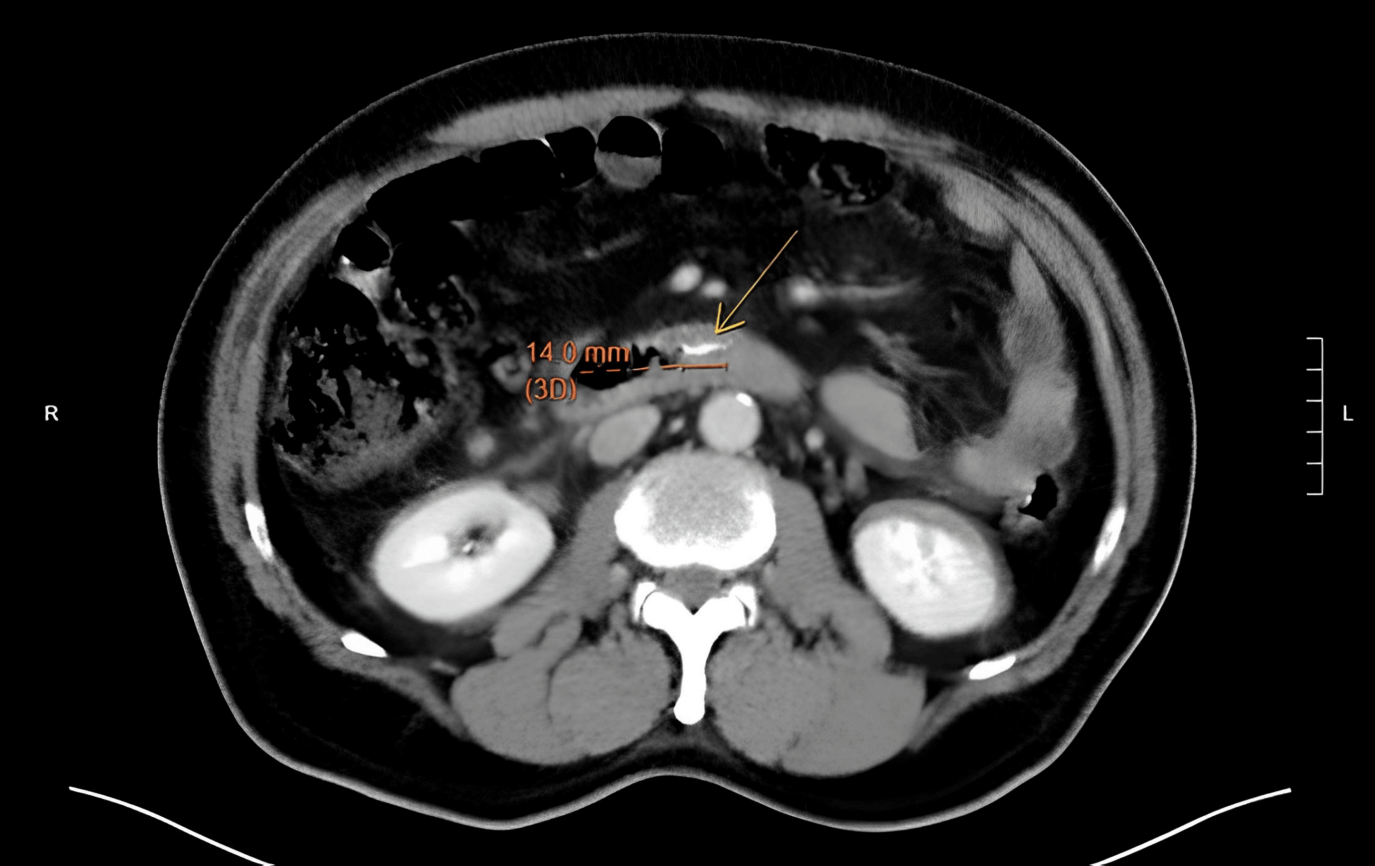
CT film showing a 1.4cm foreign body in the D3 region (annotated)
